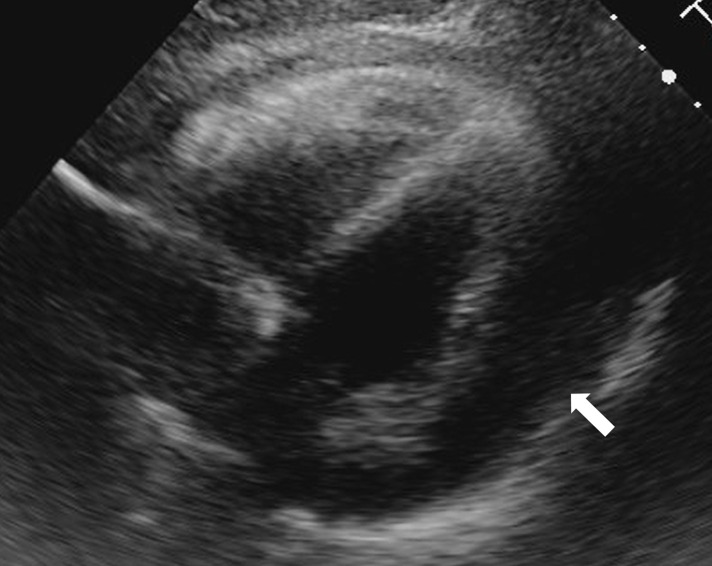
Preoperative transthoracic echocardiography showed a massive pericardial effusion (arrow).
